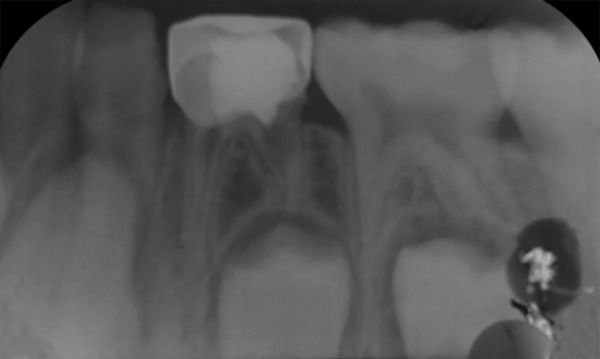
Radiographic failure in the form of internal resorption seen with turmeric pulpotomy
